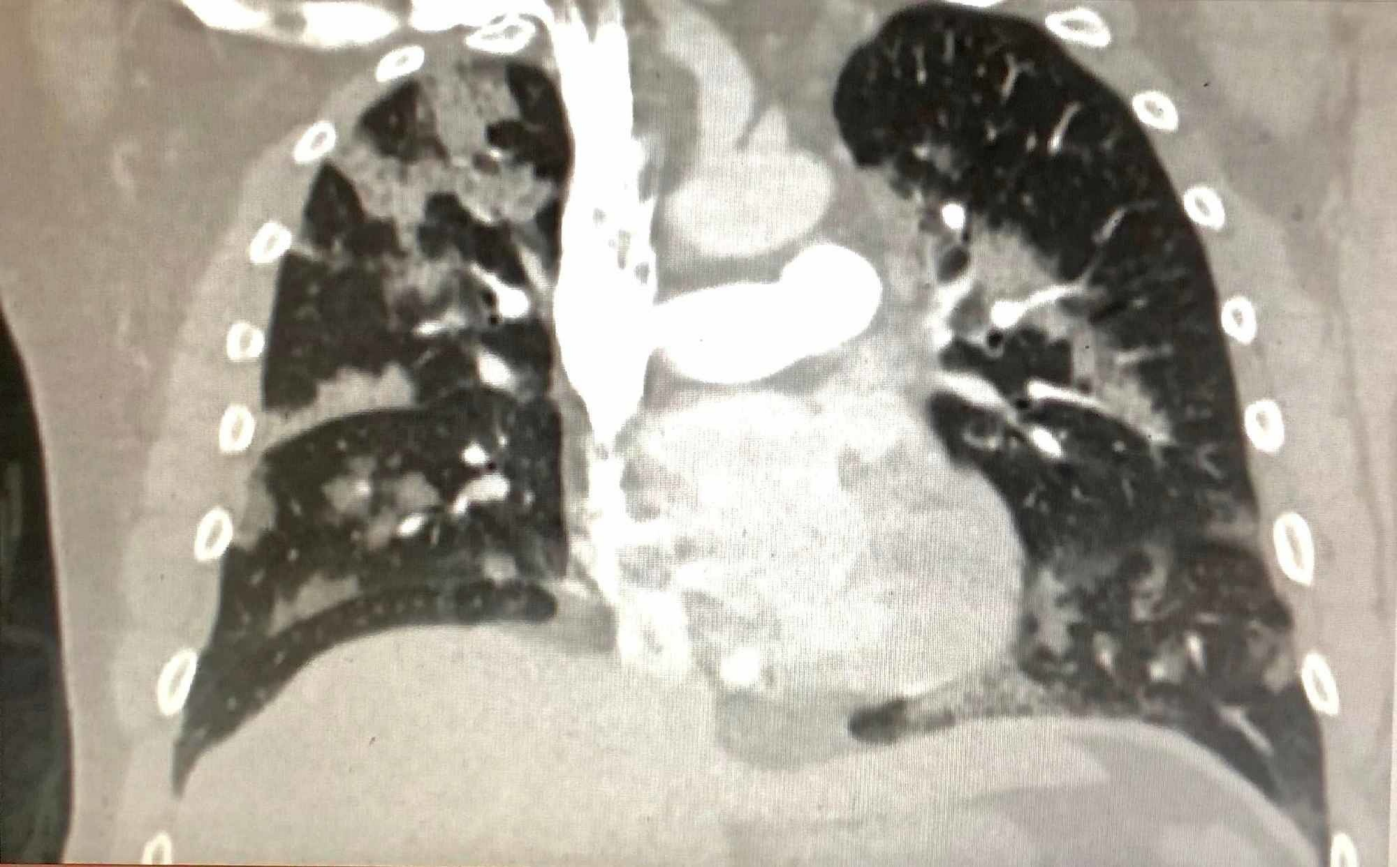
Computed tomography (CT) of the chest (coronal view) showing pulmonary edema
